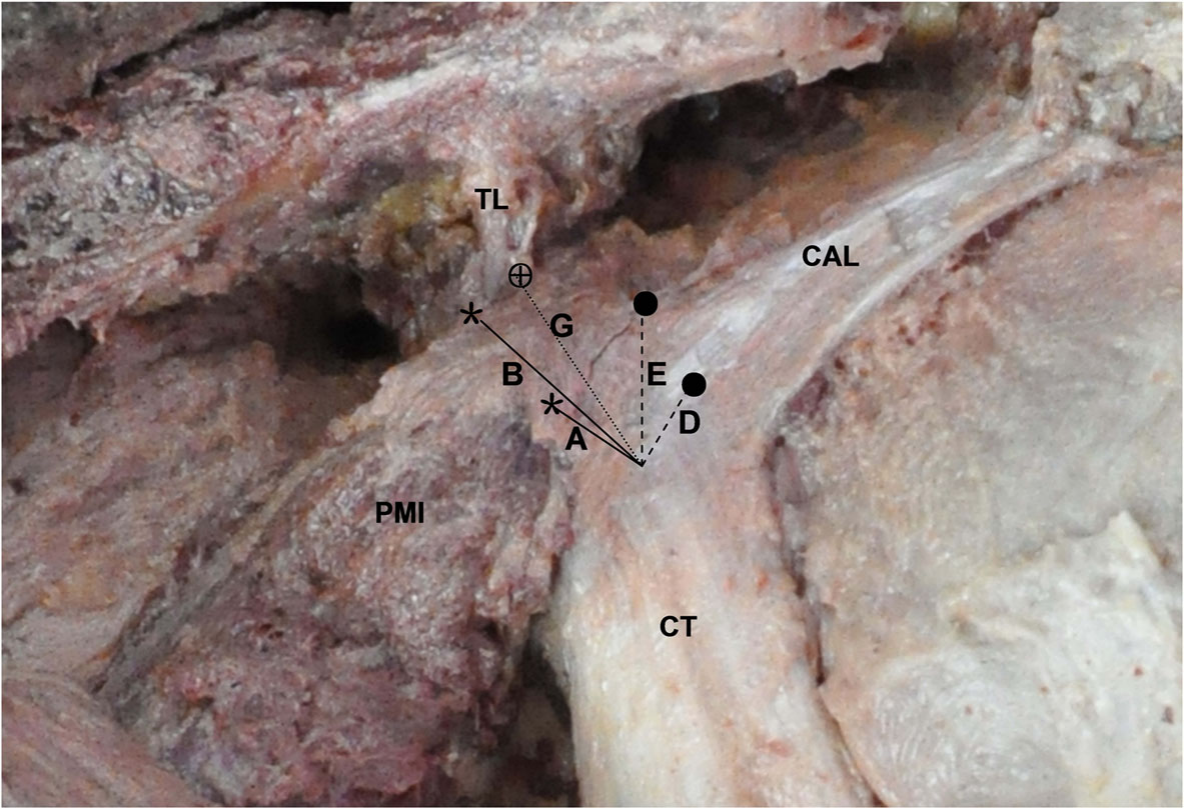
Anatomical structures of the coracoid process of the left shoulder. The *numbers* correspond to Table 1. *A*, coracoid tip to anterior pectoralis minor; *B*, coracoid tip to posterior pectoralis minor; *D*, coracoid tip to anterior coracoacromial ligament; *E*, coracoid tip to posterior coracoacromial ligament; *G*, coracoid tip to anterior coracoclavicular (C–C) trapezoid ligament (safety margin); *asterisk*, anterior and posterior margins of pectoralis minor; *filled circle*, anterior and posterior margins of coracoacromial ligament. *CAL* coracoacromial ligament, *PMI* pectoralis minor, *TL* trapezoid ligament, *CT* conjoint tendon
